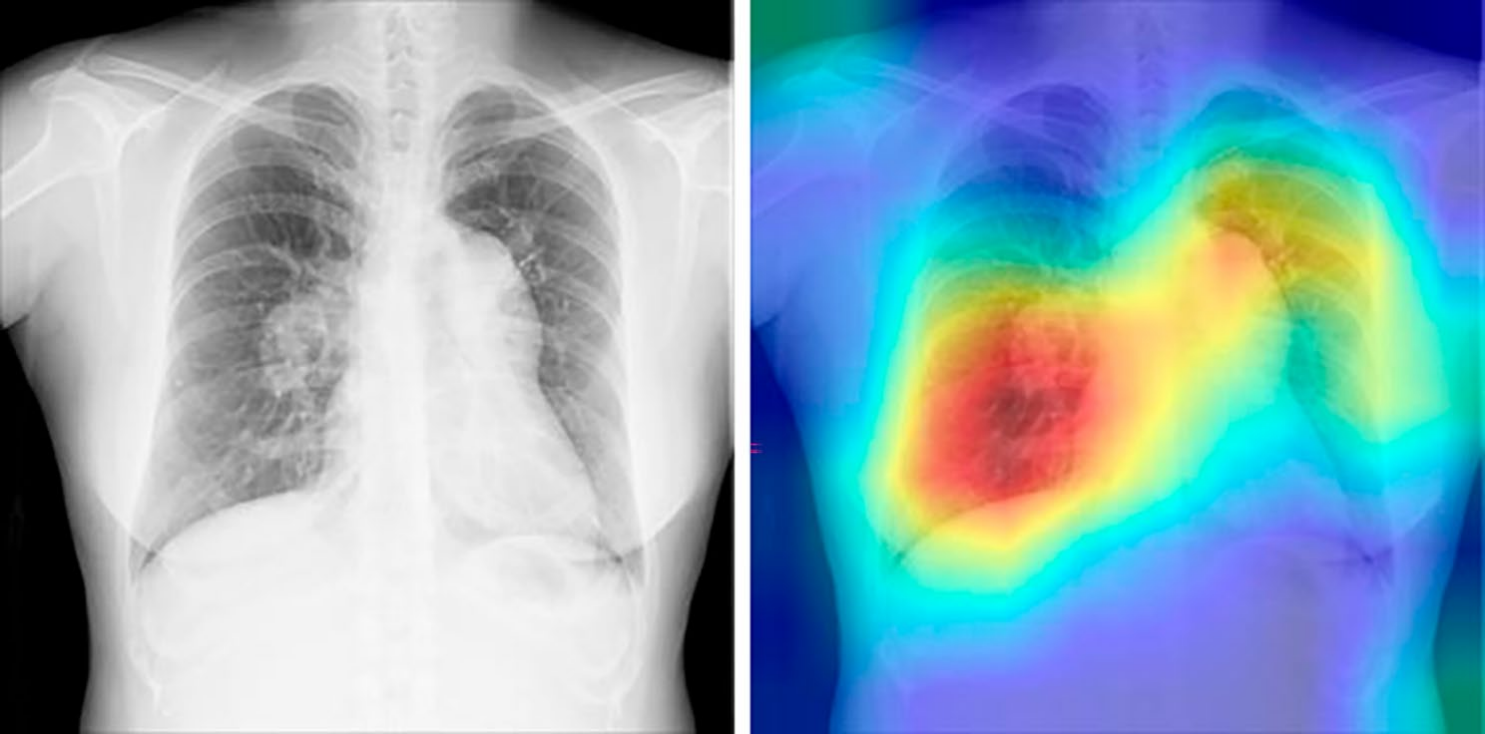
Analysis of the images where AI was focused. A single example demonstrating the function of the model. This CXR image was taken from a 39-year-old woman, with a mean pulmonary arterial pressure of 62 mmHg and WHO functional class III. Our AI model focused on the pericardial areas of the CXR images. AI: artificial intelligence; CXR: chest X-ray; WHO: World Health Organization
